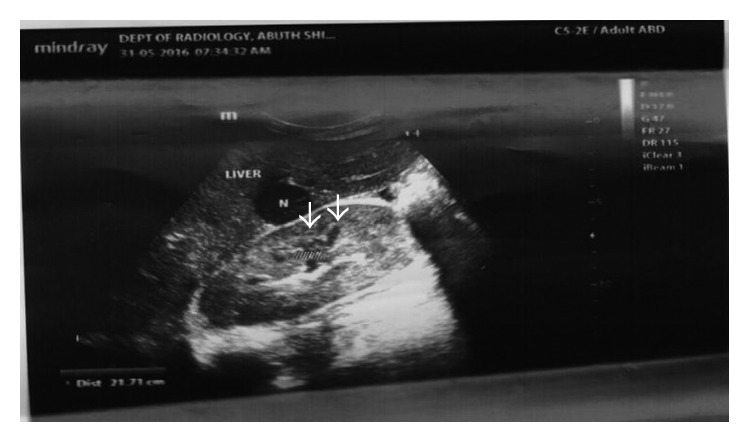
Abdominal ultrasound showing liver metastasis.
